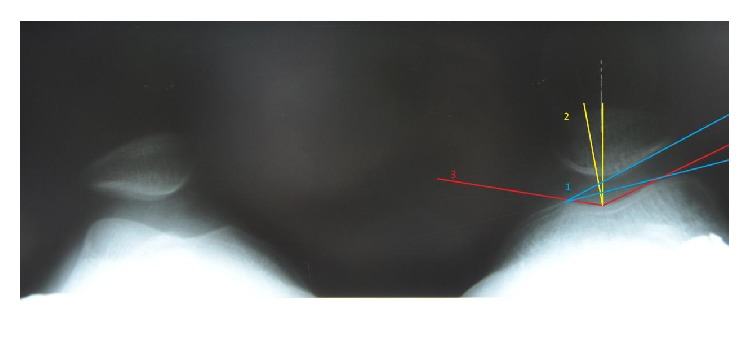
Postoperative X-rays according to Merchant view at 45° of flexion with quadriceps tension. (1) Patellofemoral angle. (2) Congruence angle. (3) Sulcus angle.
